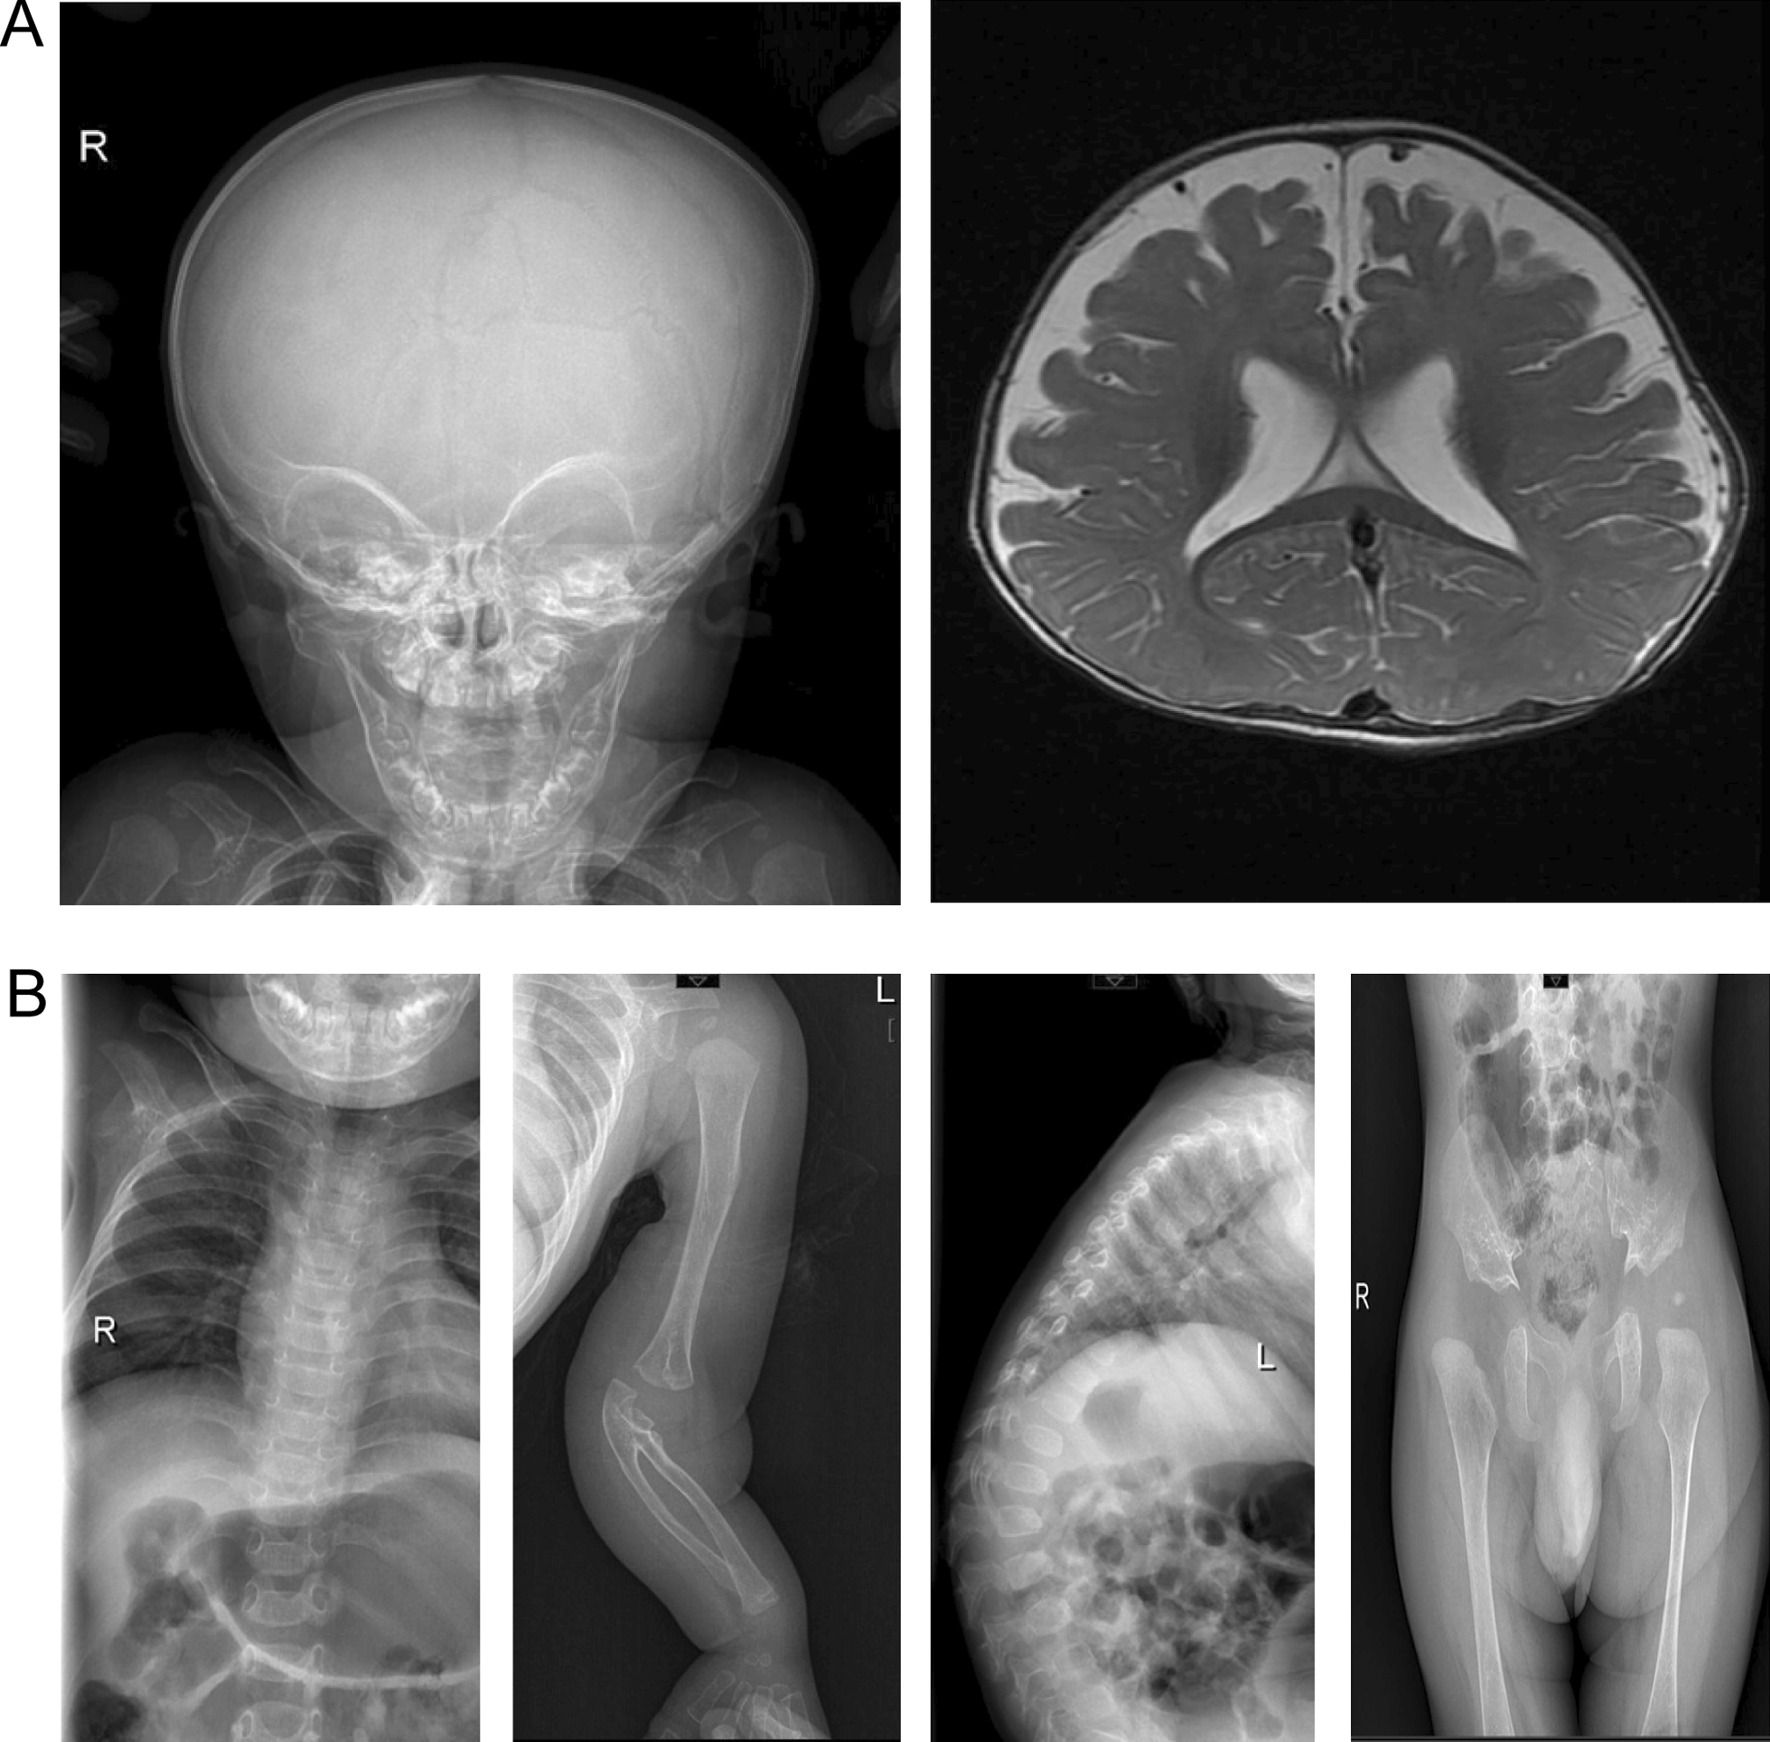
Clinical and radiological features in the present patient at the age of 7 months. Radiological features: A enlarged head, external hydrocephalus on MRI, B Scoliosis, left elbow dislocation, radioulnar synostosis, vertebral instability on T11, and T12, and bilateral dislocation of the hip
